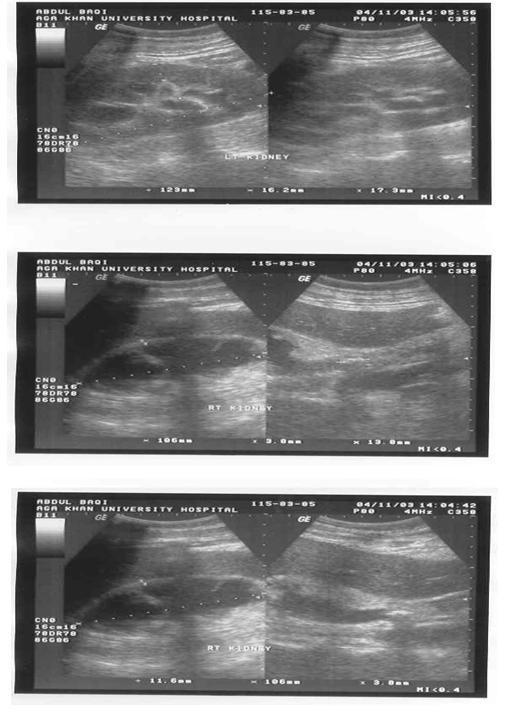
US of 65 years old male presented to emergency room with bilateral flank pain, nausea and vomiting for the past 1 week. He had an ultrasound in a peripheral hospital, which identified hydronephrosis on the right side, and percutaneous nephrostomy tube was placed. His left kidney showed hydronephrosis with renal stone (upper picture). This scan shows small-scarred right kidney (middle picture), pigtail catheter could be identified (arrow) and a proximal ureteric stone could also be seen (lower picture).
